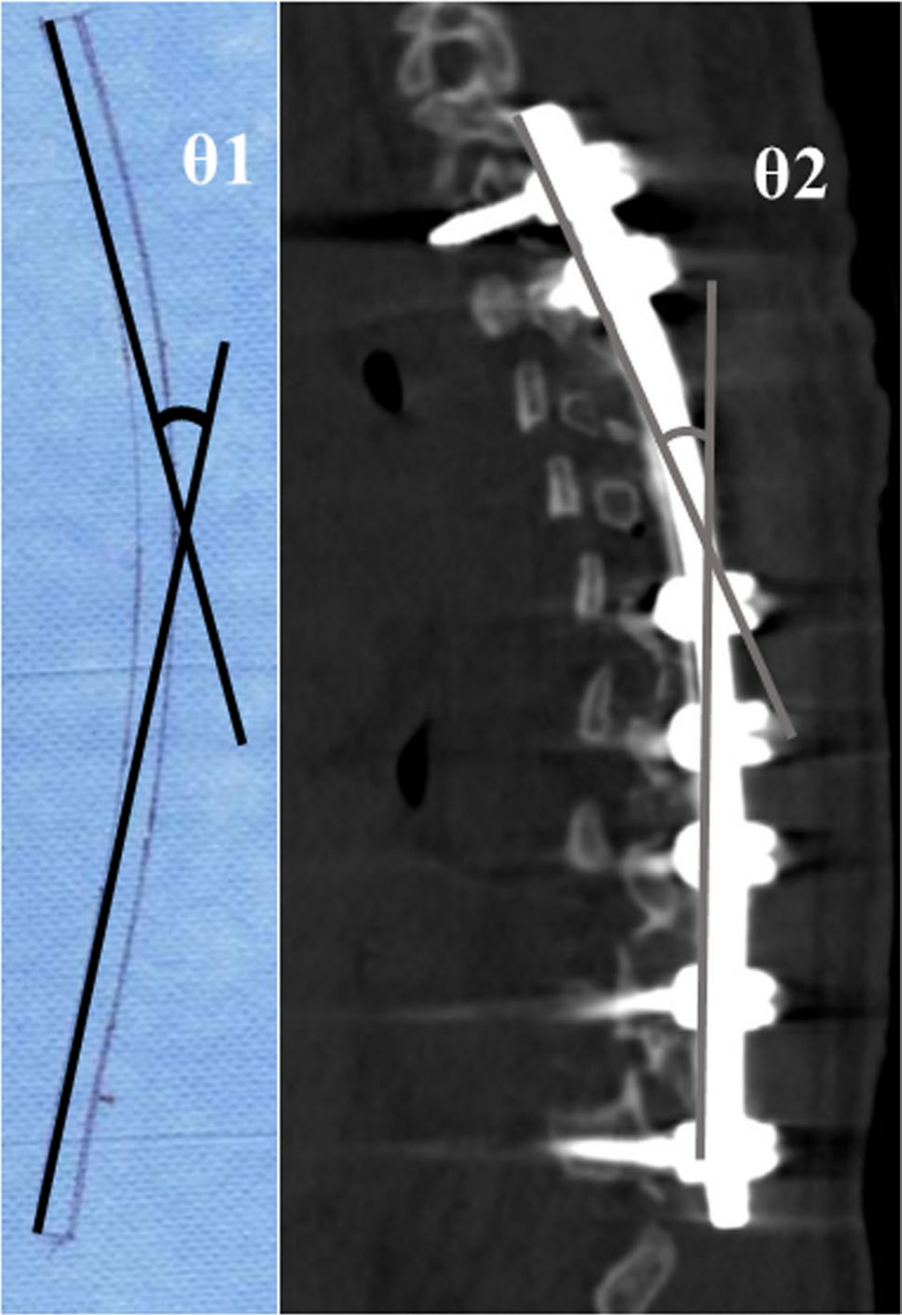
Rod angle before and after implantation. The angle between the proximal and distal tangential lines was measured. In case of notched rod, the surgeon traced the rod shapes on paper before implantation (θ1). The postoperative implant rod geometry (θ2) was obtained after the surgical operation using computed tomography.
